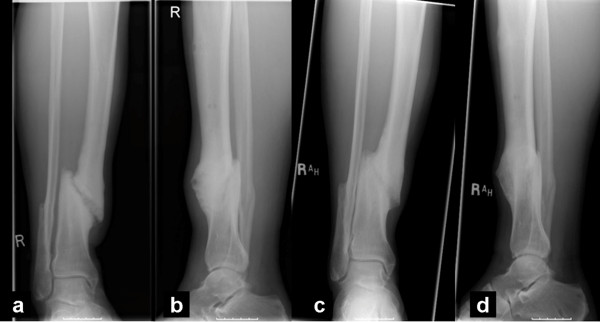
Anteroposterior (a) and lateral radiographs (b) of a distal tibial nonunion 10 months after fracture. PEMF stimulation of fracture site led to fracture union 5 months thereafter as shown in anteroposterior ( c) and lateral ( d) tibial views.
